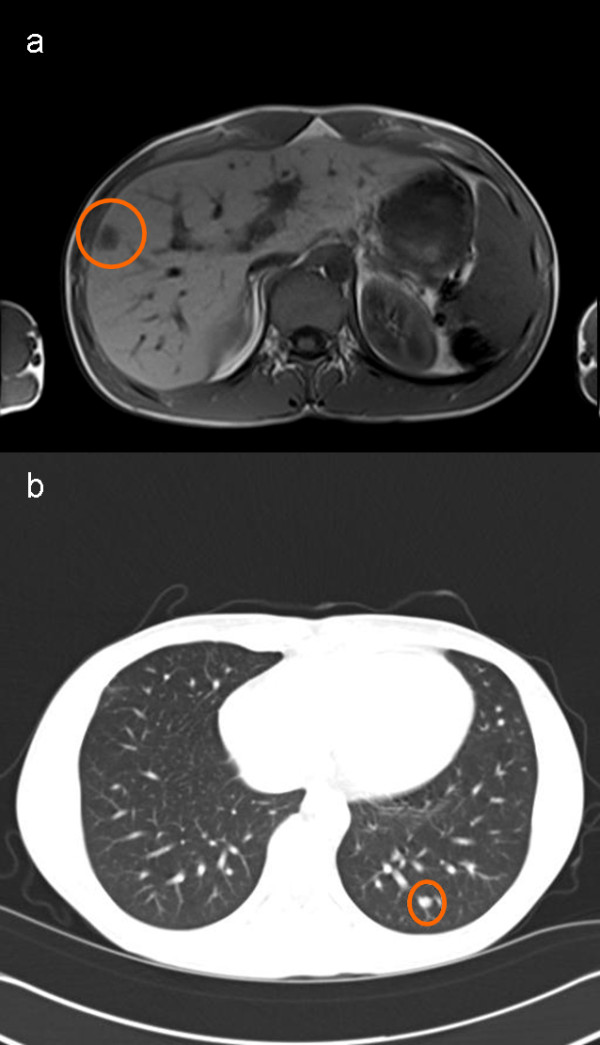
Diagnostic imaging: a) Magnetic resonance imaging (MRI) of the patient showing a colorectal liver metastasis in segment VII of the liver (*circle*) prior to its resection. b) Thoracic computed tomography exhibits a potentially metastatic, well-circumscribed lesion of 6 mm in the left lower lobe (*circle*) with homogenous contrast media enhancement. Pathological evaluation revealed a sclerosing hemangioma of the lung.
